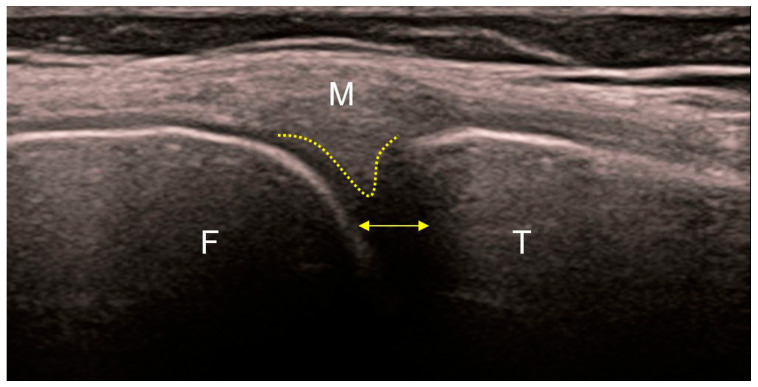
Ultrasound of the medial part of a healthy knee. Regular echoes from the femoral (F) and tibial (T) articular surfaces. The peripheral part of the medial meniscus (yellow dotted line) without a visible boundary continues into the posterior (oblique) fibers of the medial collateral ligament (M) that insert into it. The other (anterior) fibers of the medial collateral ligament continue vertically toward their insertion on the proximal tibia. Normal distance between the articular bodies indicates preserved thickness of the articular cartilages (yellow bidirectional line).
